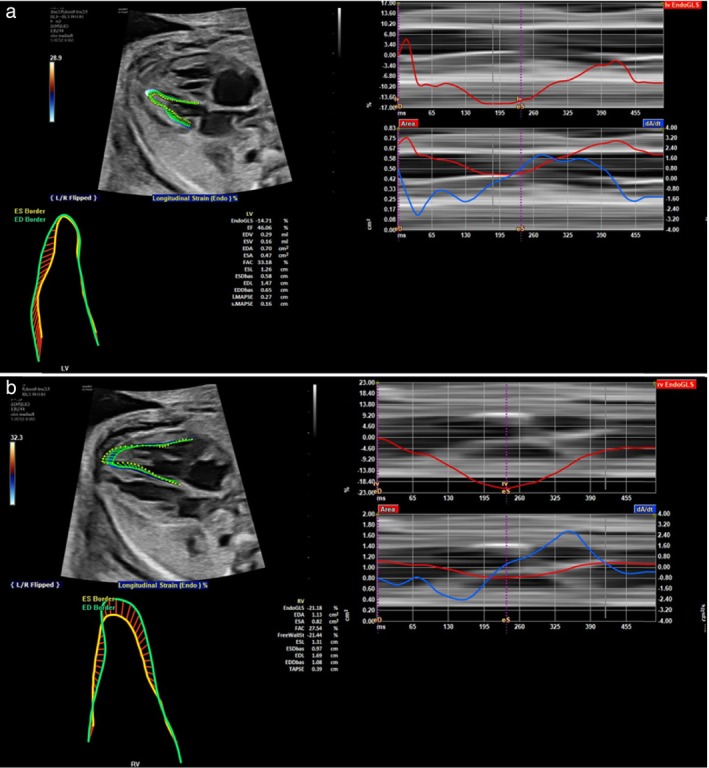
Assessment of ventricular strain. (a) Fetal left ventricular (LV) apical four‐chamber strain is derived from speckle‐tracking echocardiography using GE EchoPAC/Voluson integrated strain package. The LV endocardial border is planimetered in an apical four‐chamber view, and the myocardial ‘speckles’ are then tracked throughout systole and diastole with generation of tracings and measurement of strain and strain rate. The graph shown is a strain curve, demonstrating smooth systolic deformation with normal peak systolic strain followed by diastolic relaxation. Importantly, assessment of strain requires high frame rates to generate accurate measures and may differ between different software. (b) Similarly, the right ventricular strain and strain rate can be assessed. ED Border, end‐diastolic endocardial border; ES Border, end‐systolic endocardial border.
